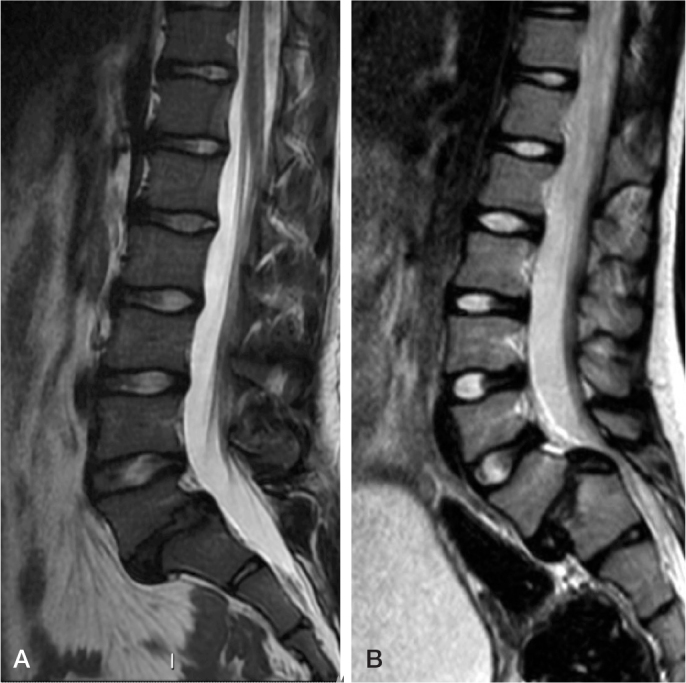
(A) Isthmic and (B) dysplastic spondylolisthesis in T2 weighted MR images. Central spinal canal remains wide in the isthmic lesion, while in the dysplastic lesion it has narrowed. The sacrum has developed a ridge necessitating sacral osteotomy (ridge resection) to facilitate reduction of the forward slip and bony healing.
